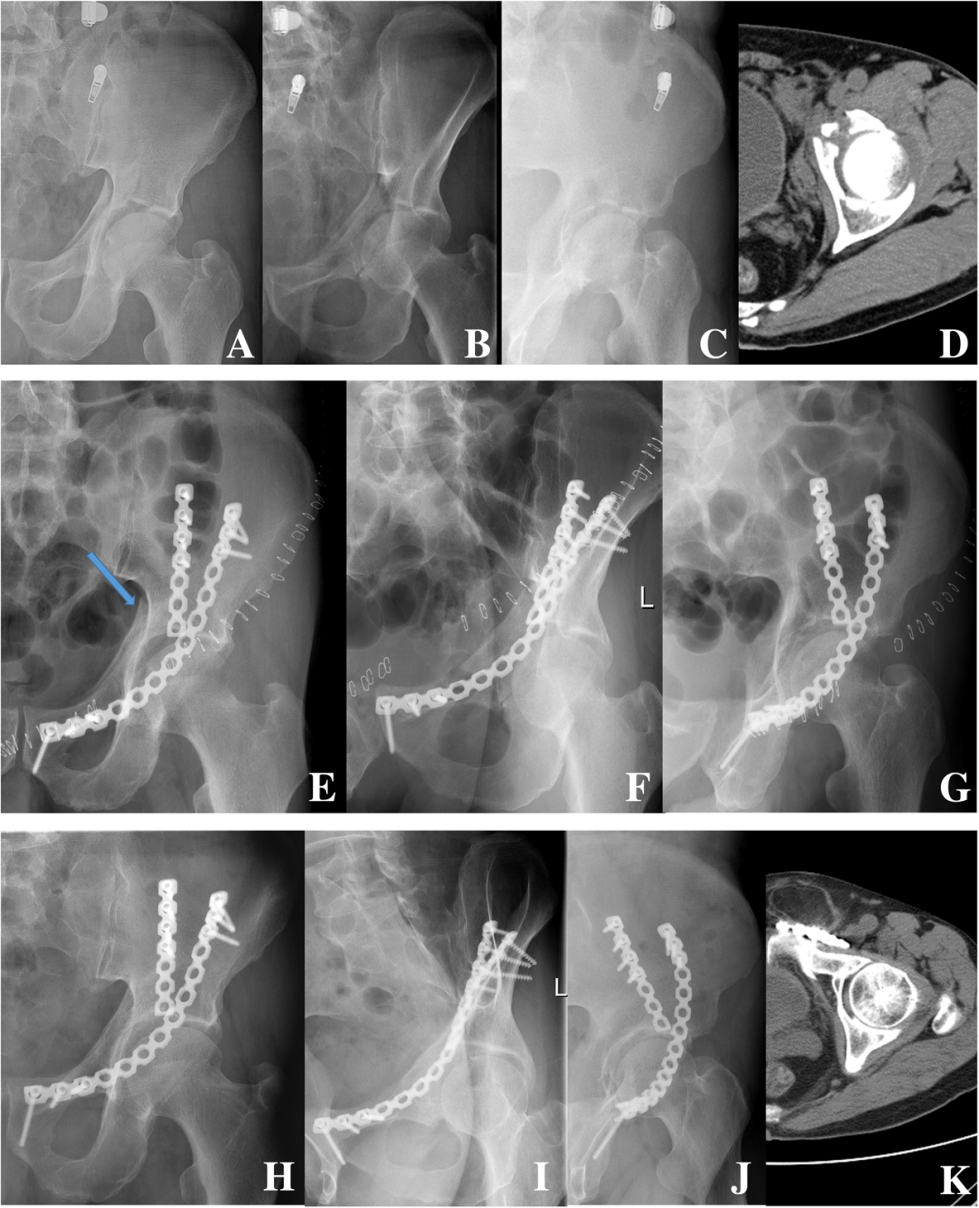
Radiographs of a 75-year-old male patient with an anterior wall acetabular fracture. a Anteroposterior radiograph; b Obturator oblique radiograph; c Iliac oblique radiograph; d CT scan. Radiographs showing imperfect reduction (arrow) of the fracture immediately after the operation. e Anteroposterior radiograph; f Obturator oblique radiograph; g Iliac oblique radiograph. Radiographs showing an excellent radiological outcome at 84 months after the operation and the clinical score was 18 points. h Anteroposterior radiograph; i Obturator oblique radiograph; j iliac oblique radiograph; k CT scan
